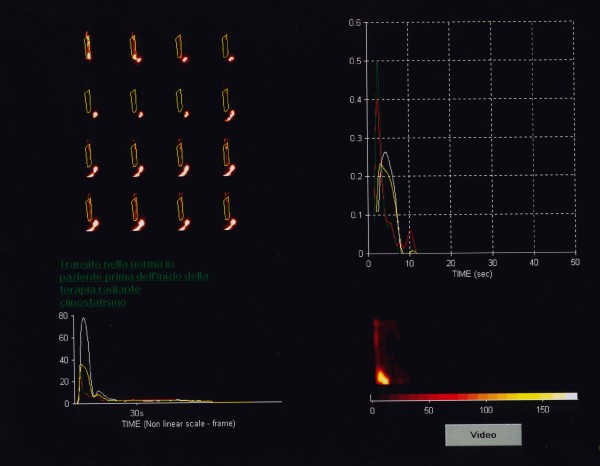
Oesophageal scintigraphy: Dynamic condensed oesophageal images before radiation therapy (normal transit).
